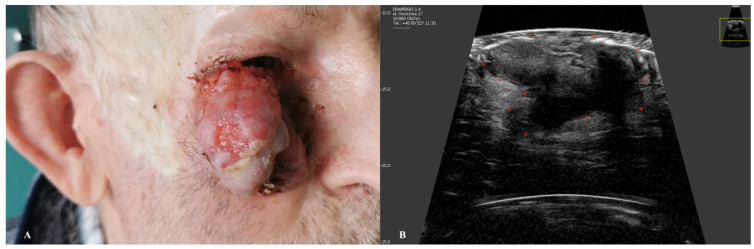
Macroscopic image of Merkel cell carcinoma (A) with a corresponding ultrasonographic image (B). Stars indicate tumor margins. The yellow box indicates the area covered by the ultrasonographic field of view.
